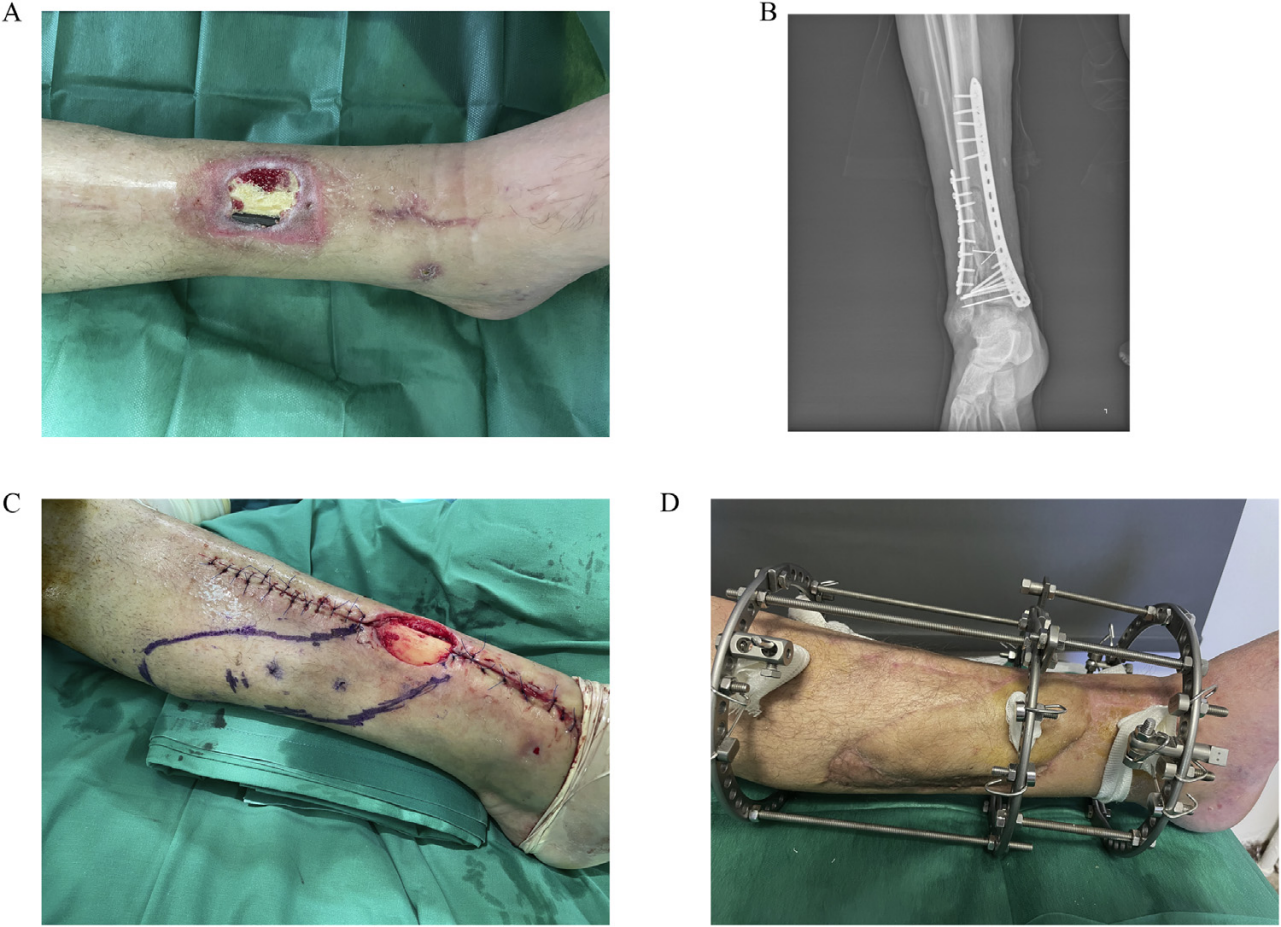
(A) A 74 year-old patient with wound dehiscence and implant exposure over the distal third of his anterior tibia. (B) X-ray of intra-articular distal tibia and fibula fracture stabilized with plates and screws. (C) Full-thickness defect with tibia exposure following surgical debridement and removal of hardware. Design of a local propeller flap to cover the defect. (D) Satisfactory wound healing after flap reconstruction and application of Ilizarov framework.
